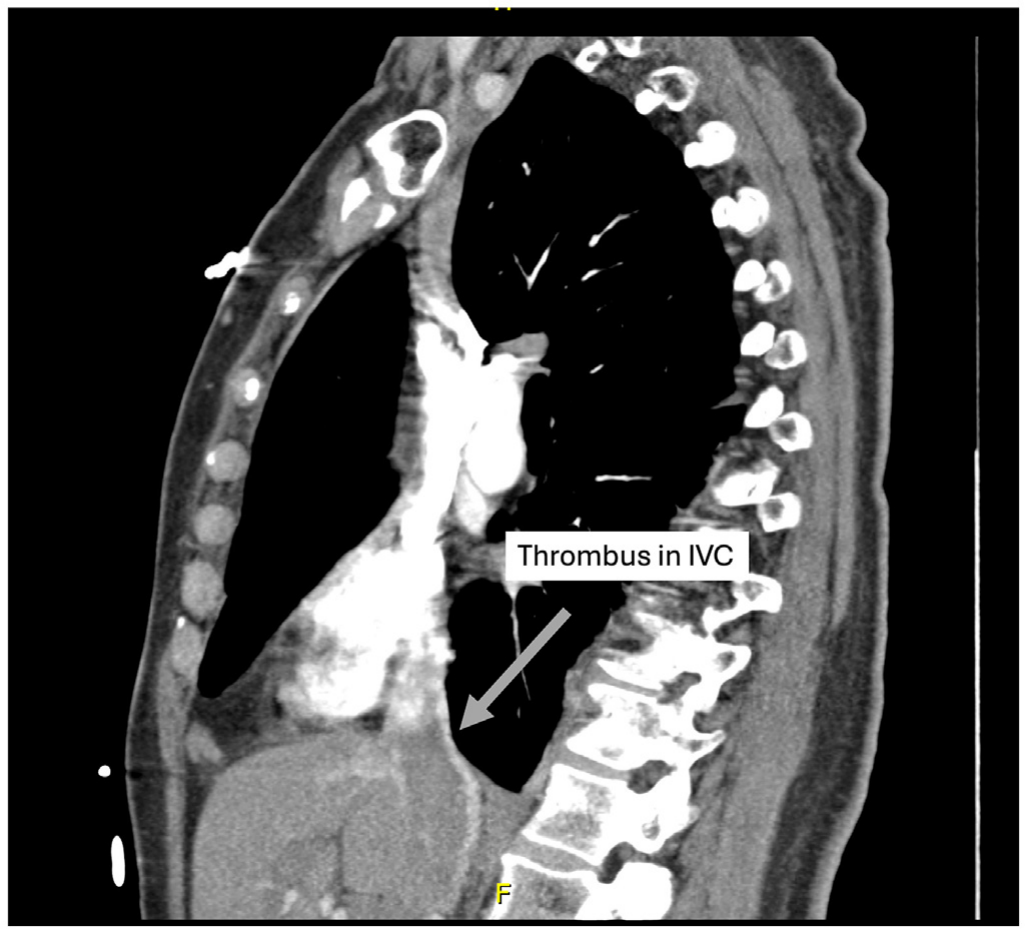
Computed tomography angiogram demonstrating thrombus in the supradiaphragmatic inferior vena cava (*IVC*).
